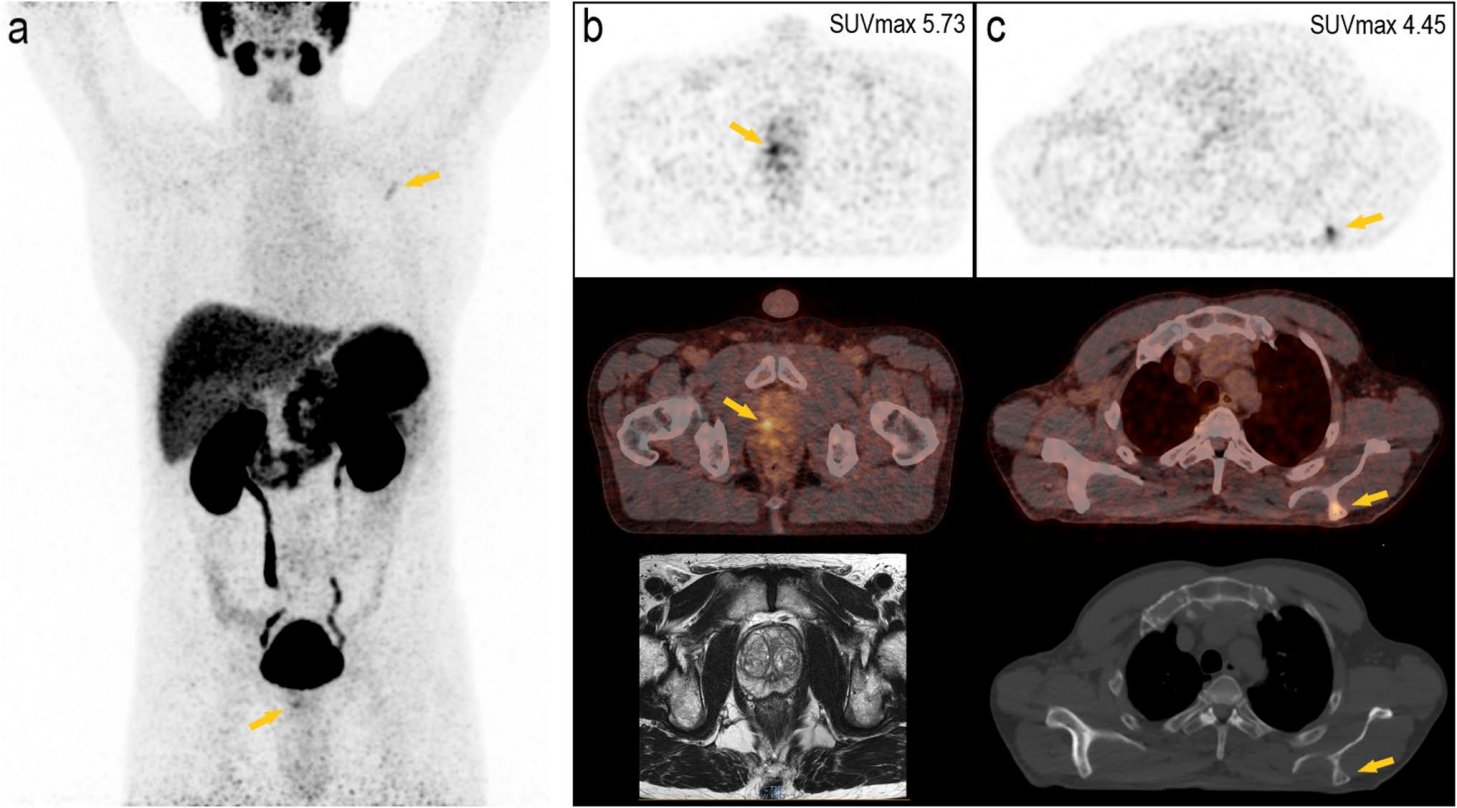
The additive value of [^68^ Ga]Ga-PSMA-11 PET/CT in the detection of distant bone metastases. A 58-year-old intermediate-risk prostate cancer patient, Gleason score 7a, and tPSA of 19.08 ng/ml showed suspicious focal uptake on the left spine of the scapula without morphological changes on CT. a Maximum intensity projection image; b index lesion on PET imaging, index lesion on fused PET/CT imaging, and T2-weighted MRI (PIRADS 3); c bone lesion on PET imaging, bone lesion on fused PET/CT imaging, and CT image in bone window.
